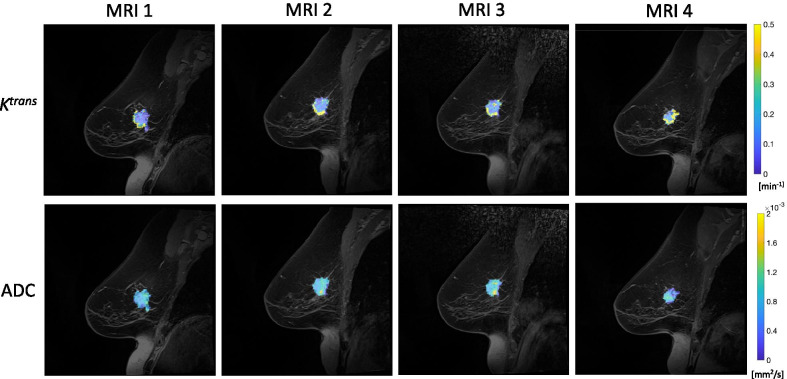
Representative *K*^trans^ (top) and ADC (bottom) maps over the course of therapy for a woman who had stable disease (patient #4 in Table 1). Median *K*^trans^ values increase over the course of therapy, from 0.10 min^−1^ at MRI 1 to 0.15 min^−1^ at MRI 2 and to 0.14 min^−1^ at MRIs 3 and 4. Mean tumor ADC values are largely unchanged over the course of therapy, from 0.0009 mm^2^/s at MRIs 1, 2, and 3 to 0.0008 mm^2^/s at MRI 4. For display purposes, *K*^trans^ and ADC parametric maps are interpolated to the resolution of anatomical images and overlaid on top of the anatomical images. (Note that all analysis was performed on the resolution at which the data were acquired.)
